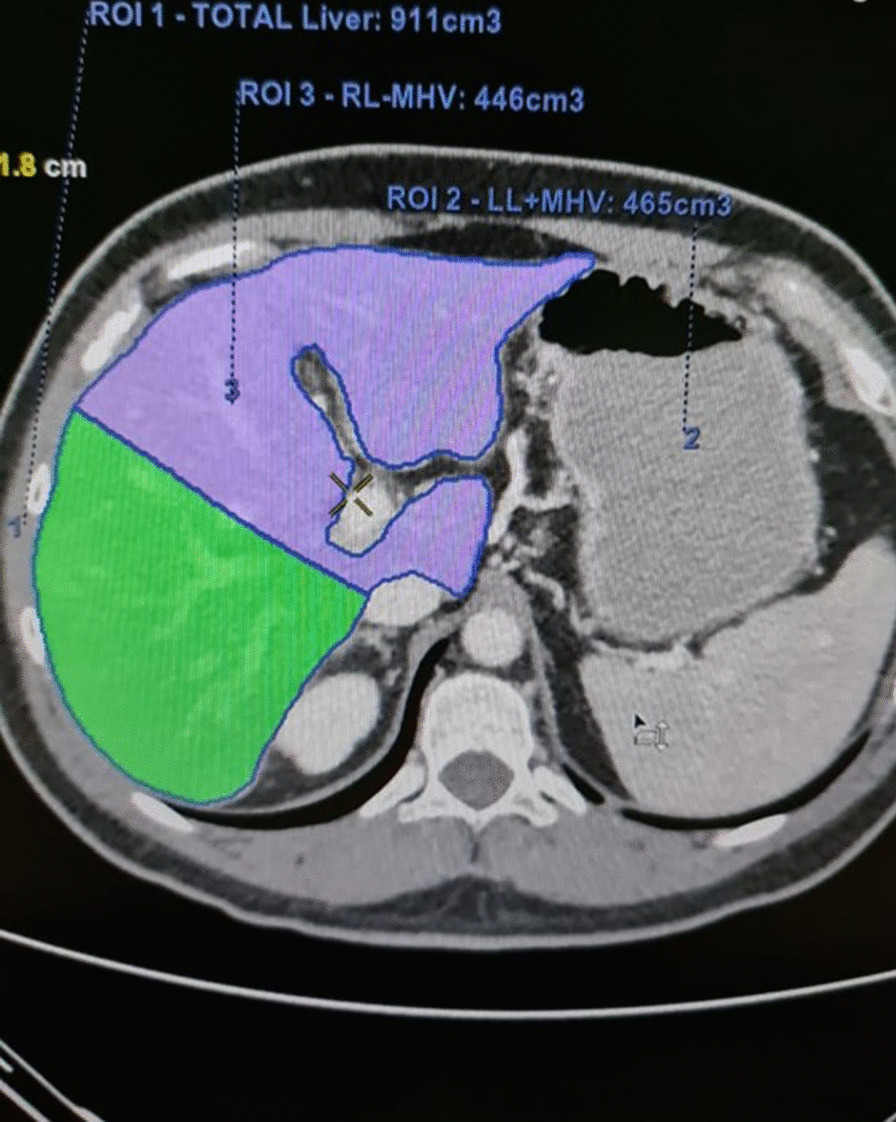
CT of the abdomen with volumetry showing an estimated volume of the possible future liver remnant (left liver lobe) of approximately 51%
